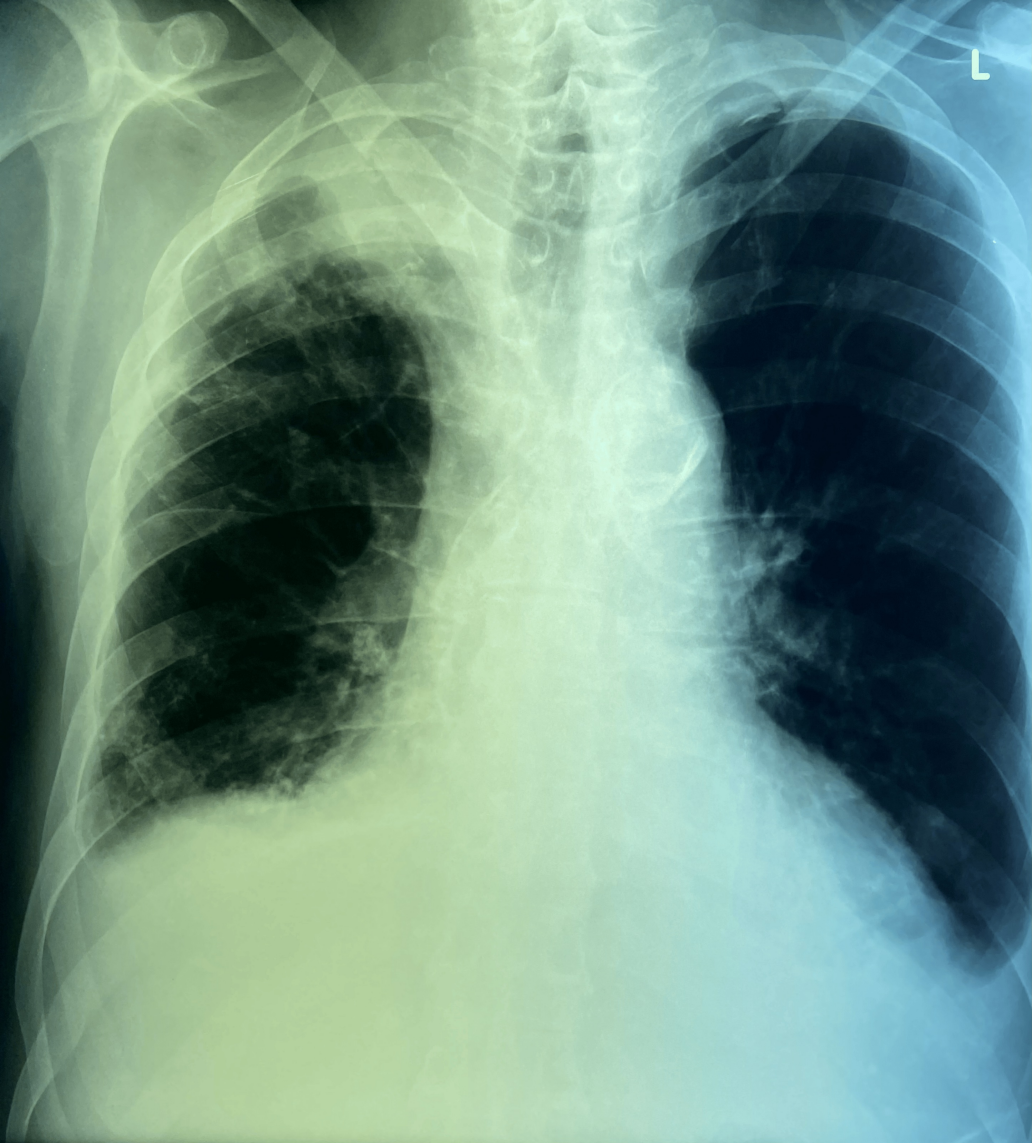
Anterior-posterior view chest X-ray showing regression of pneumonia.
